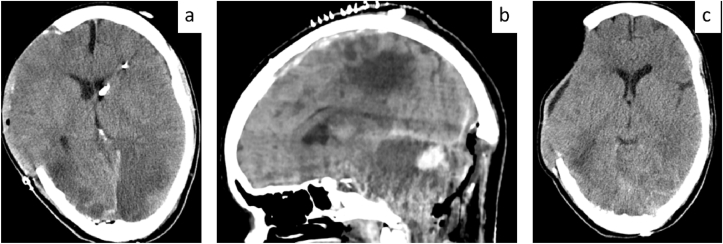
Representative Case 4: Severe wound healing complication after decompressive craniectomy – primary LPS implantation. 5a. Axial cranial CT scan following right-sided decompressive hemicraniectomy and EVD placement in a patient with severe TBI. 5b. Sagittal CT scan after posterior fossa decompression due to uncontrollable intracranial hypertension and transtentorial herniation. 5c. Axial CT scan after LPS implantation demonstrating stable postsurgical conditions without signs of CSF accumulation or midline shift.
